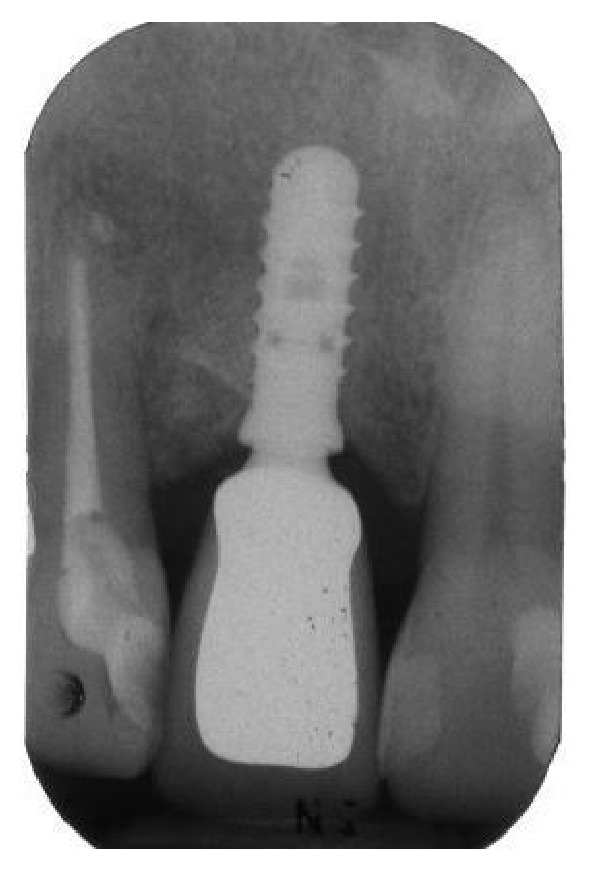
Periapical radiograph taken two years after implant placement.
